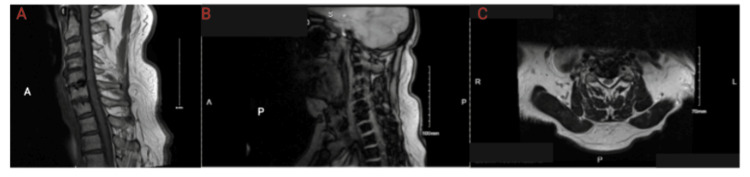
Cervical MRI Imaging Images (A-C) highlight the areas of osteophytic changes including multilevel vertebral space narrowing, central canal and foraminal narrowing, and disc osteophytic complexes.
